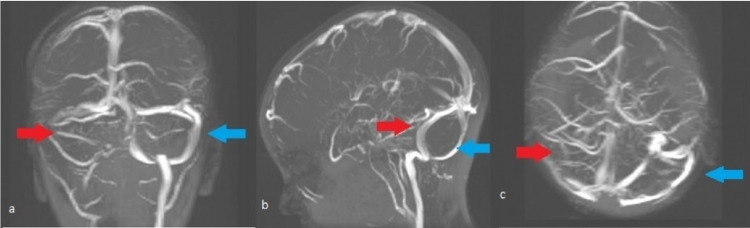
MRI-MRV cross-sections (a. coronal, b. sagittal, c. transverse) After the administration of a paramagnetic substance, a filling defect is noted in the final part of the right internal jugular vein and the sigmoid sinus on the same side. (Red arrow depicts the filling defect, Blue arrow depicts the normal contralateral side)
